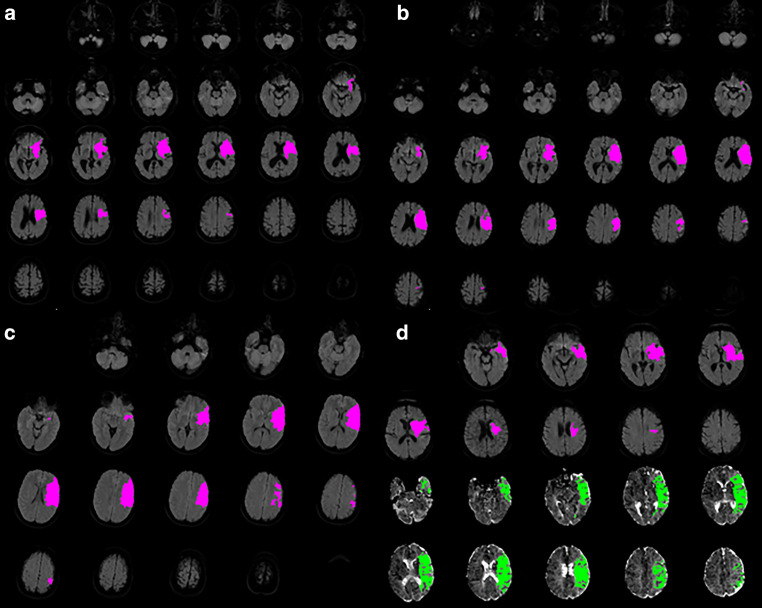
Representation of RAPID cut-offs used to compare the long-term outcome after mechanical thrombectomy according to baseline advanced imaging characteristics. (a, b, c) represent infarct core volumes of approximately 50 ml, 70 ml respectively 100 ml (*pink areas*) assessed here on baseline MRI using diffusion-weighted-imaging. (d) represent an absolute mismatch of approximately 50 ml assessed as volume with Tmax > 6 s on perfusion imaging (*green areas*) minus volume with ischemic core volume (*pink areas*)
